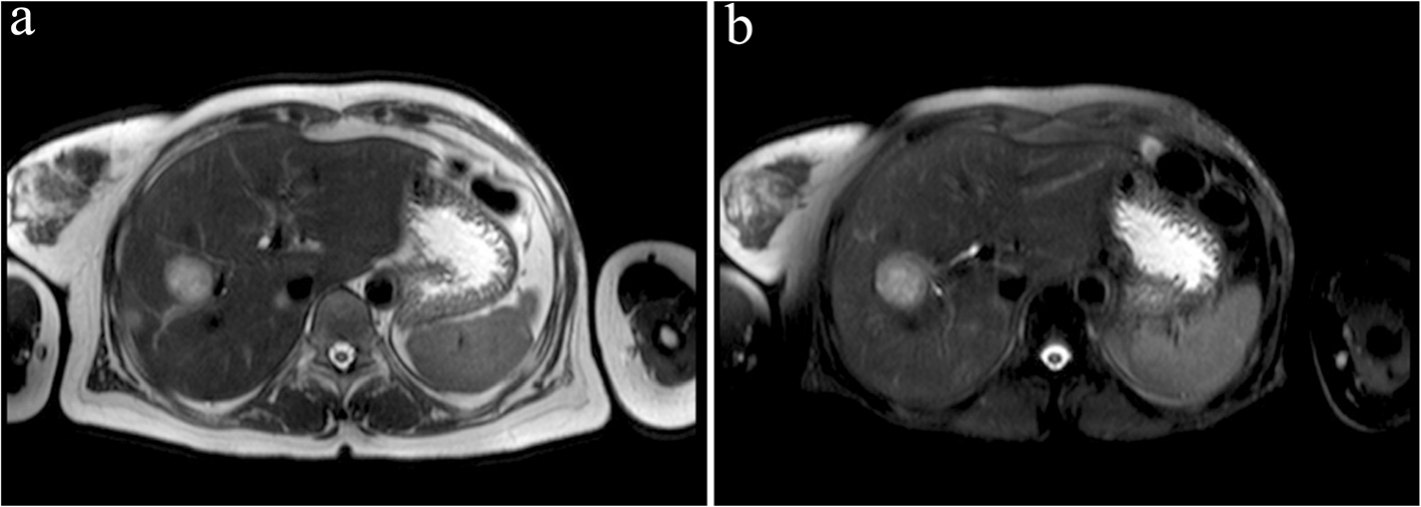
Liver magnetic resonance image (MRI) after the patient have continued irregular fever for 1 month
